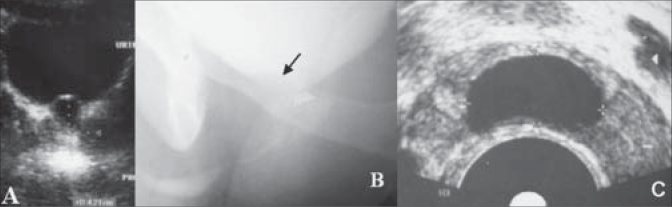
(A) Abdominal ultrasound, (B) VCUG and (C) TRUS image of midline prostatic cyst
